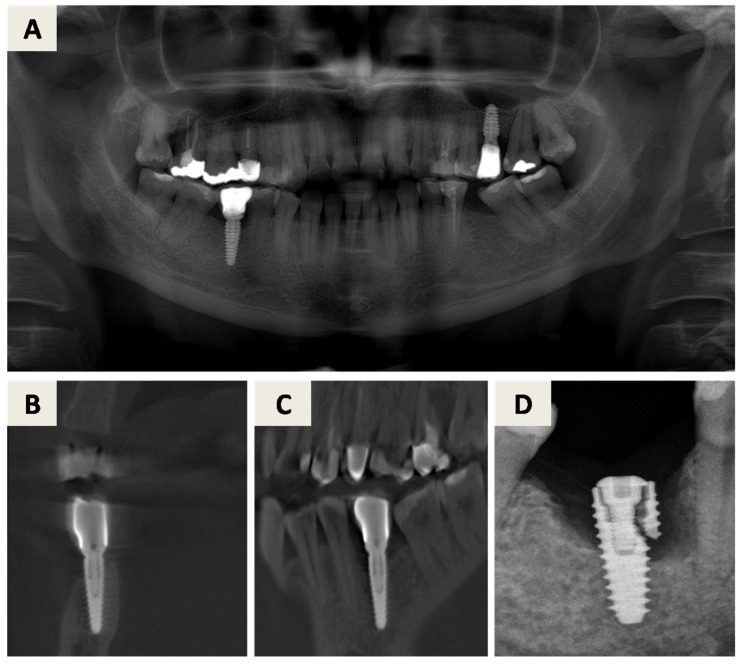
(A) Orthopantomogram with visible peri-implant lesion around the implant in position 46. (B,C) Cone-beam computed tomography (CBCT) images in coronal (B) and sagittal (C) planes taken in the pre-surgical phase showing a horizontal defect (Class II of the Monje classification of peri-implant bone defects); (D) RTG taken right before surgical procedure, demonstrating fractured implant in position 46.
